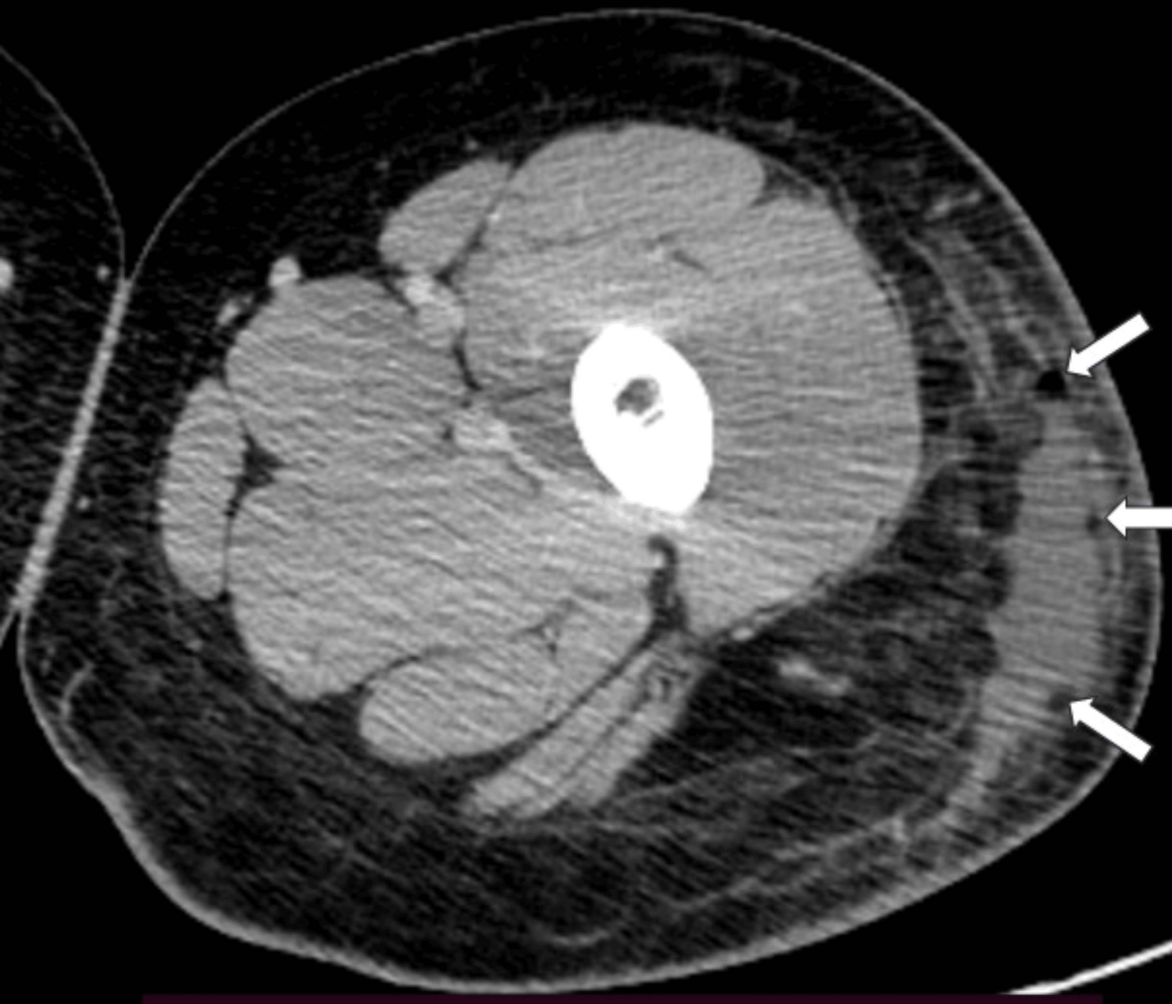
CT scan of the left thigh. CT scan with contrast imaging (2.5 mm slices) revealed an irregular crescent-shaped fluid collection and small amounts of gas (arrows) in the soft tissues of the left lateral thigh.
